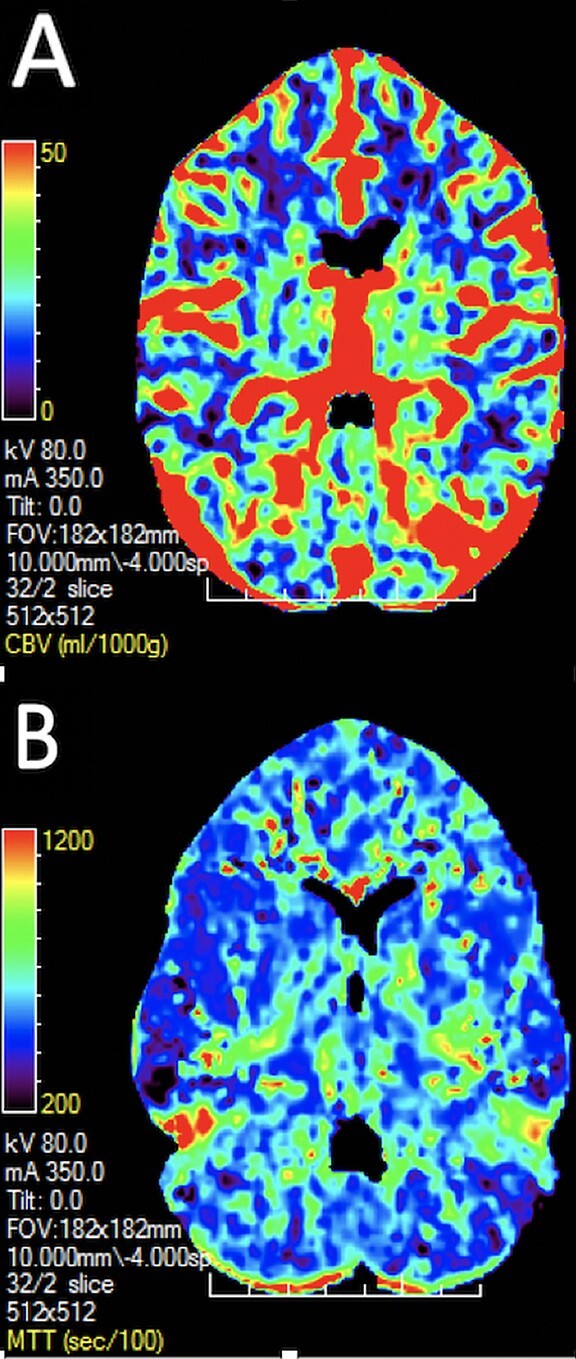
Images A and B show the CT perfusion map appearances of cerebral blood volume and mean transit time, respectively. Image A shows a normal and symmetrical appearance of cerebral blood volume (a measure of the volume of blood (ml) in a given amount of brain tissue (1000g)). Image B shows a normal and symmetrical appearance of mean transit time (a measure of how quickly blood is moving through the brain). Note, in both images, the grey and white matter of the brain return different signals owing to their different perfusion parameters.
